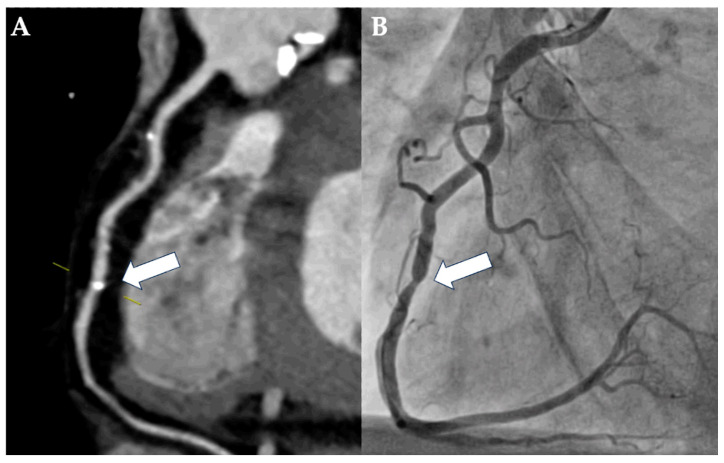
Case example of a rare false-negative assessment of pre-TAVI-CT. A 58-year-old man with severe AS was evaluated for TAVI due to hostile chest and chronic obstructive pulmonary disease with long-term oxygen therapy. Imaging was performed using first-generation dual-source CT and was of good quality despite minor streak artifacts arising from contrast material in the right ventricle. Based on the vessel view of the right coronary artery (A), the localized calcification of the mid segment was assessed as being mild (25–49% luminal diameter stenosis; CAD-RADS 2; white arrow). However, ICA revealed a severe, ca. 80% luminal diameter stenosis of the corresponding lesion in the mid-segment of the right coronary artery ((B); white arrow).
